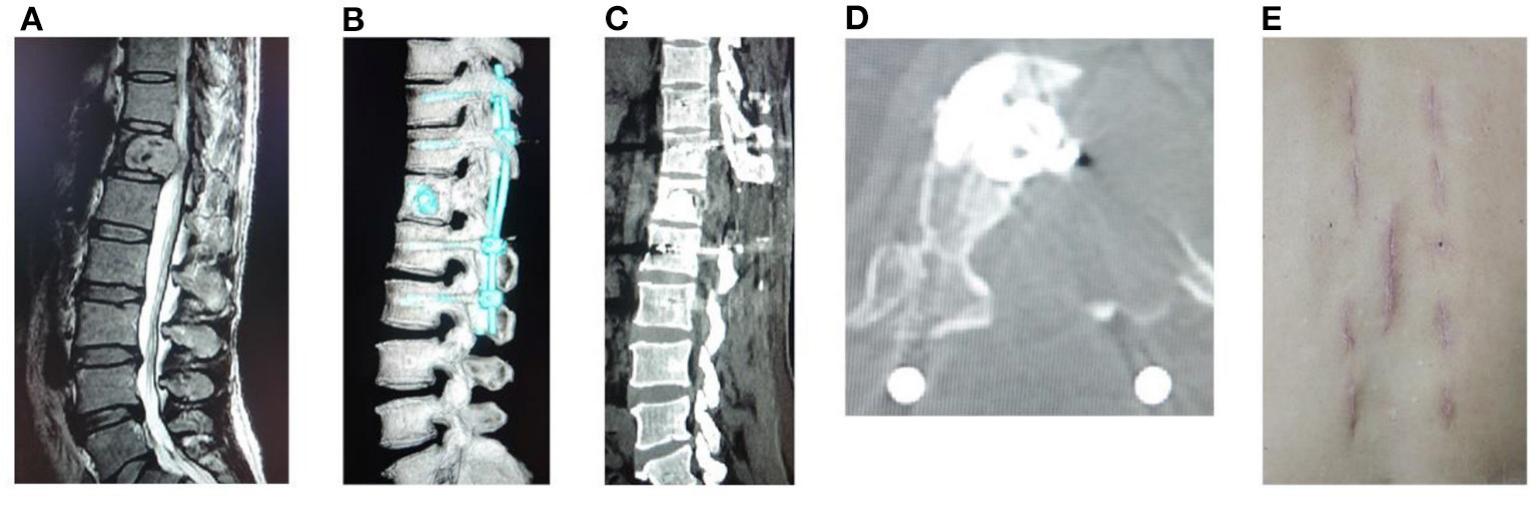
A male patient of 67 years old with T12 metastasis and neurologic compression from HCC had back pain VAS 9, ASIA B, Tomita 6, modified Tokuhashi 11, ESCC grade 3, and SINS 8. He underwent freehand MIPS+PVP, minimally invasive decompression, and partial tumor resection, with an operation duration of 215 min, intraoperative blood loss of 90 ml, incision length of 3.5 cm, and stay in hospital of 5 days. (A) Sagittal MRI showed metastasis of T12 with neurologic compression. (B) Sagittal CT reconstruction. (C,D) Sagittal and axial CT showed complete decompression and no leakage of cement into the spinal canal. (E) Incision after minimally invasive surgery.
